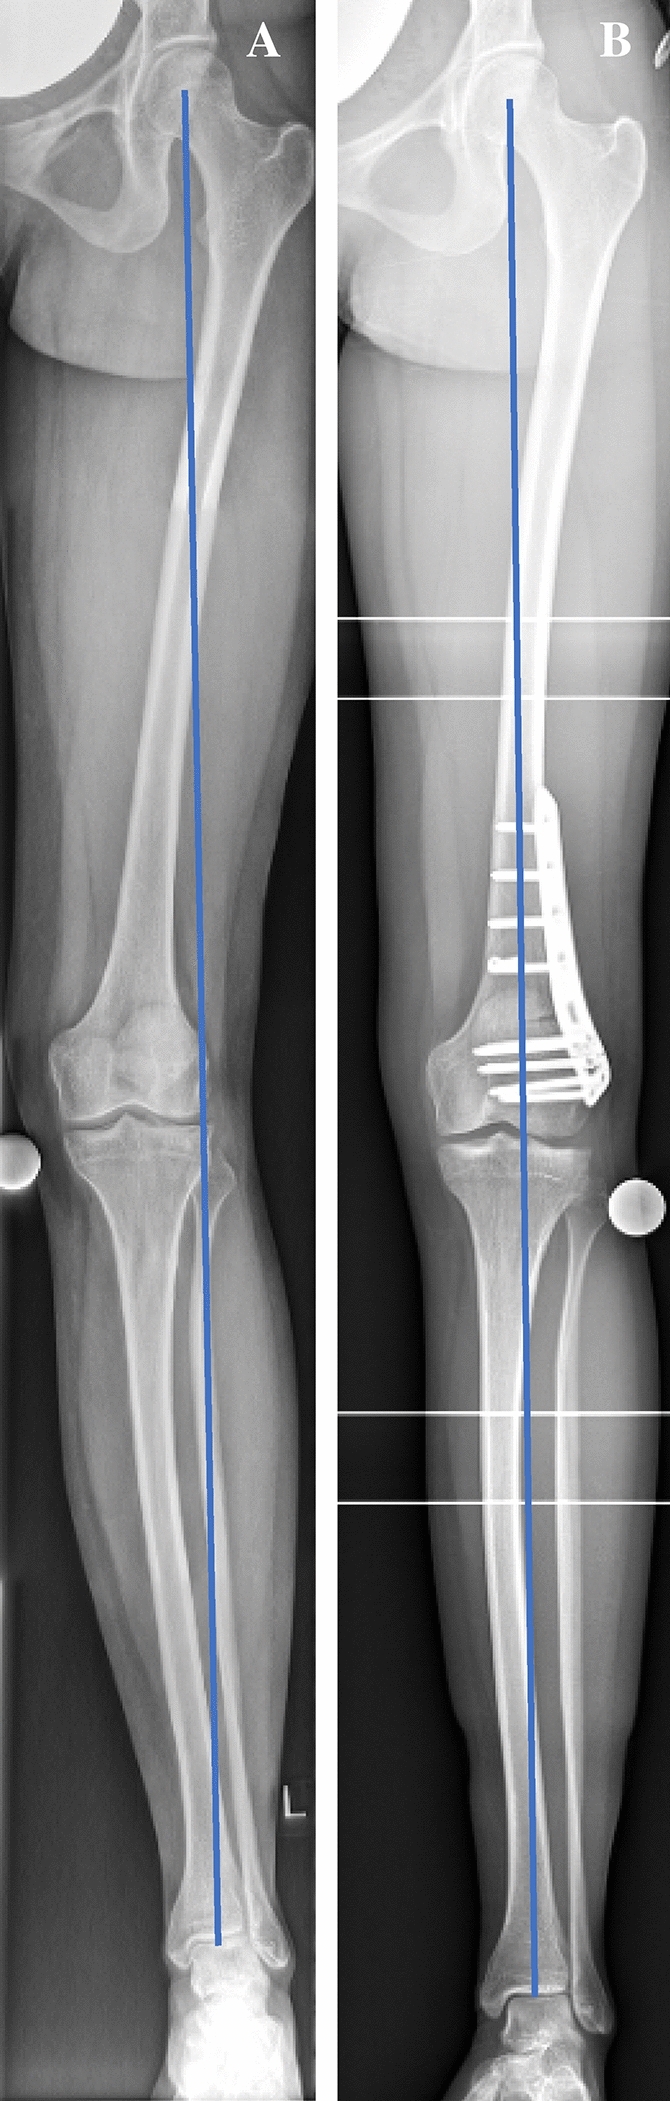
Weight-bearing whole-leg anteroposterior radiographs taken pre- (A) and postoperatively (B) following a valgus-correcting osteotomy
